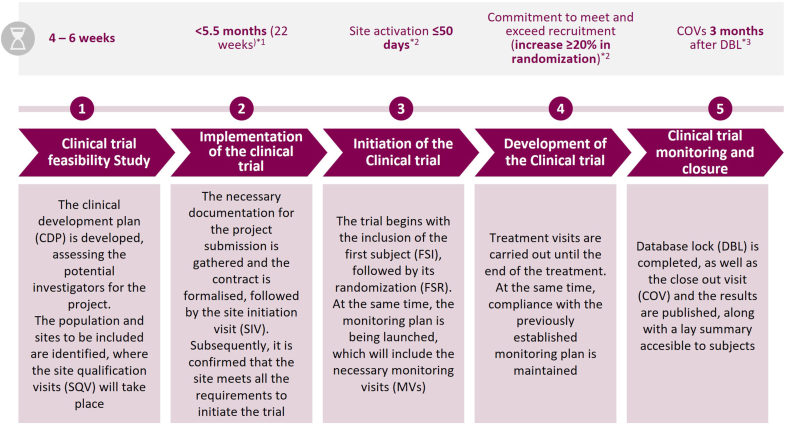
The “ideal” process for the development of a CT. ∗^1^Based on EU Clinical Trials Register experience throughout 2024 [2,12,16]. CT: clinical trial. [1] Regulatory harmonisation of clinical trials in the EU: Clinical Trials Regulation to enter into application and new Clinical Trials Information System to be launched. Available at: https://www.ema.europa.eu/en/news/regulatory-harmonisation-clinical-trials-eu-clinical-trials-regulation-enter-application-and-new-clinical-trials-information-system-be-launched. [2] European Medicines Agency. Clinical Trials in the European Union. Available in: Clinical Trials in the European Union – EMA https://euclinicaltrials.eu/. [3] ICH HARMONISED GUIDELINE GUIDELINE FOR GOOD CLINICAL PRACTICE E6(R3). INTERNATIONAL COUNCIL FOR HARMONISATION OF TECHNICAL REQUIREMENTS FOR PHARMACEUTICALS FOR HUMAN USE. Final version Adopted on January 06, 2025. Available at: https://database.ich.org/sites/default/files/ICH_E6%28R3%29_Step4_FinalGuideline_2025_0106.pdf.
